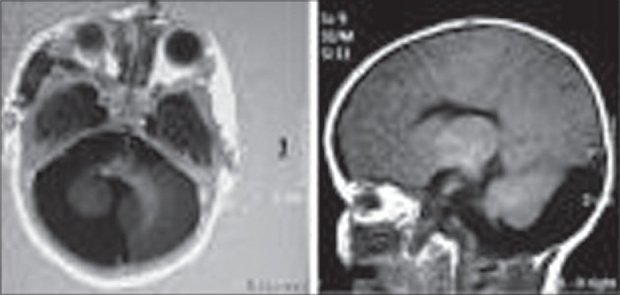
(T1 Weighted Images of MRI Brain): Dandy Walker Cyst Associated with Hypoplastic Vermis and Right Cerebellar Hemisphere
